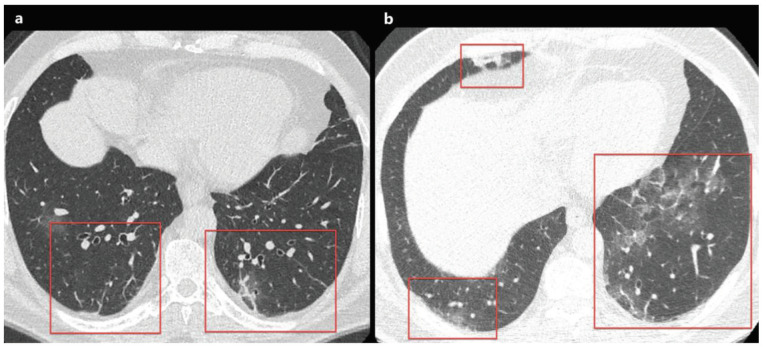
a) A 66-year-old symptomatic man with COVID-19. Axial CT image shows subpleural fibrous stripes with ground-glass opacities in both lower lobes (red frames). b) A 53-year-old man with COVID-19. Axial CT image shows subpleural curvilinear lines, interlobular septal thickening, and fibrous stripes with ground-glass opacities in both lower lobes (red frames).
